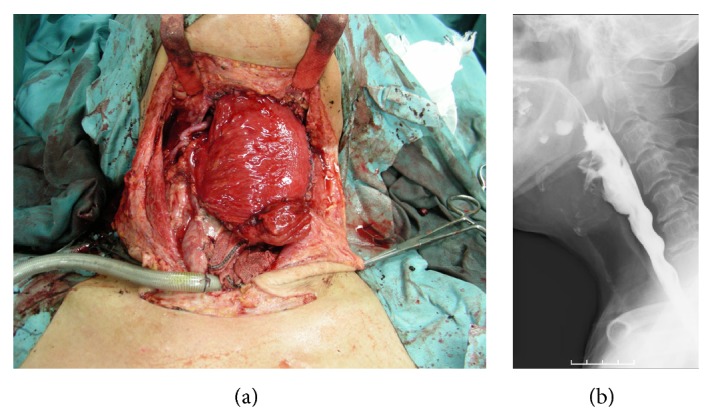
(a) The second jejunal vessels were anastomosed to lingual artery and internal jugular vein with vein grafts. (b) The postoperative X-ray finding of swallowing. There were no leakage and stenosis.
